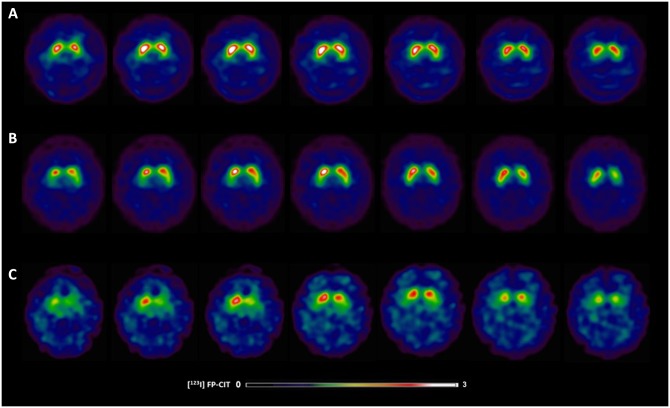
[^123^I]FP-CIT SPECT images in Parkinson’s disease patients with and without dysphagia. (A) 63-year-old healthy control showing typical [^123^I]FP-CIT specific binding ratios in the caudate (SBR: 3.87) and putamen (SBR: 2.65) (B) 63-year-old male without swallowing difficulties exhibiting slight dopaminergic deficits as reflected by [^123^I]FP-CIT specific binding ratios in the caudate (SBR: 2.92) and putamen (SBR: 2.15); (C) 63-year-old female with swallowing difficulties demonstrating larger striatal dopaminergic deficits as reflected by [^123^I]FP-CIT specific binding ratios in the caudate (SBR: 1.00) and putamen (SBR: 0.58).
